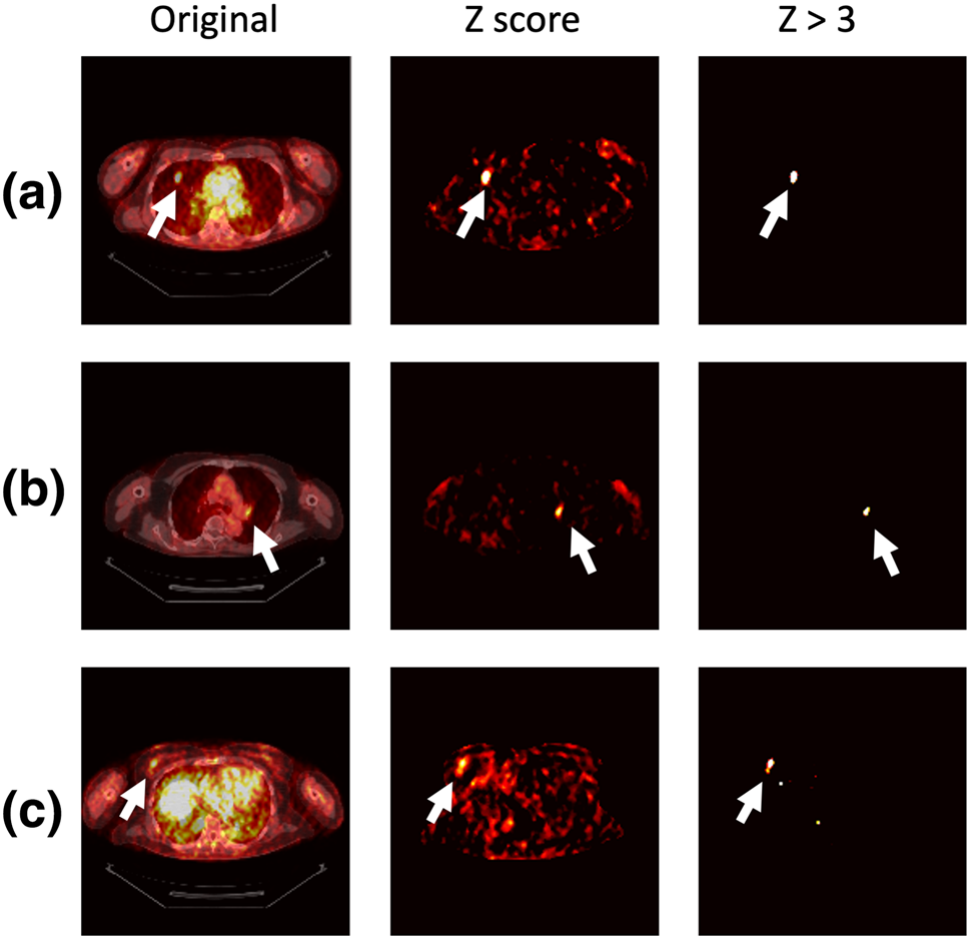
Examples of images for our anomaly detection. The original images (fused PET/CT) and Z-score maps obtained by the proposed method are shown in the left and middle columns, respectively. The images in the right column show the regions with a Z-score greater than 3. a Lung mass. b Left hilar lymph node. c Right breast mass
